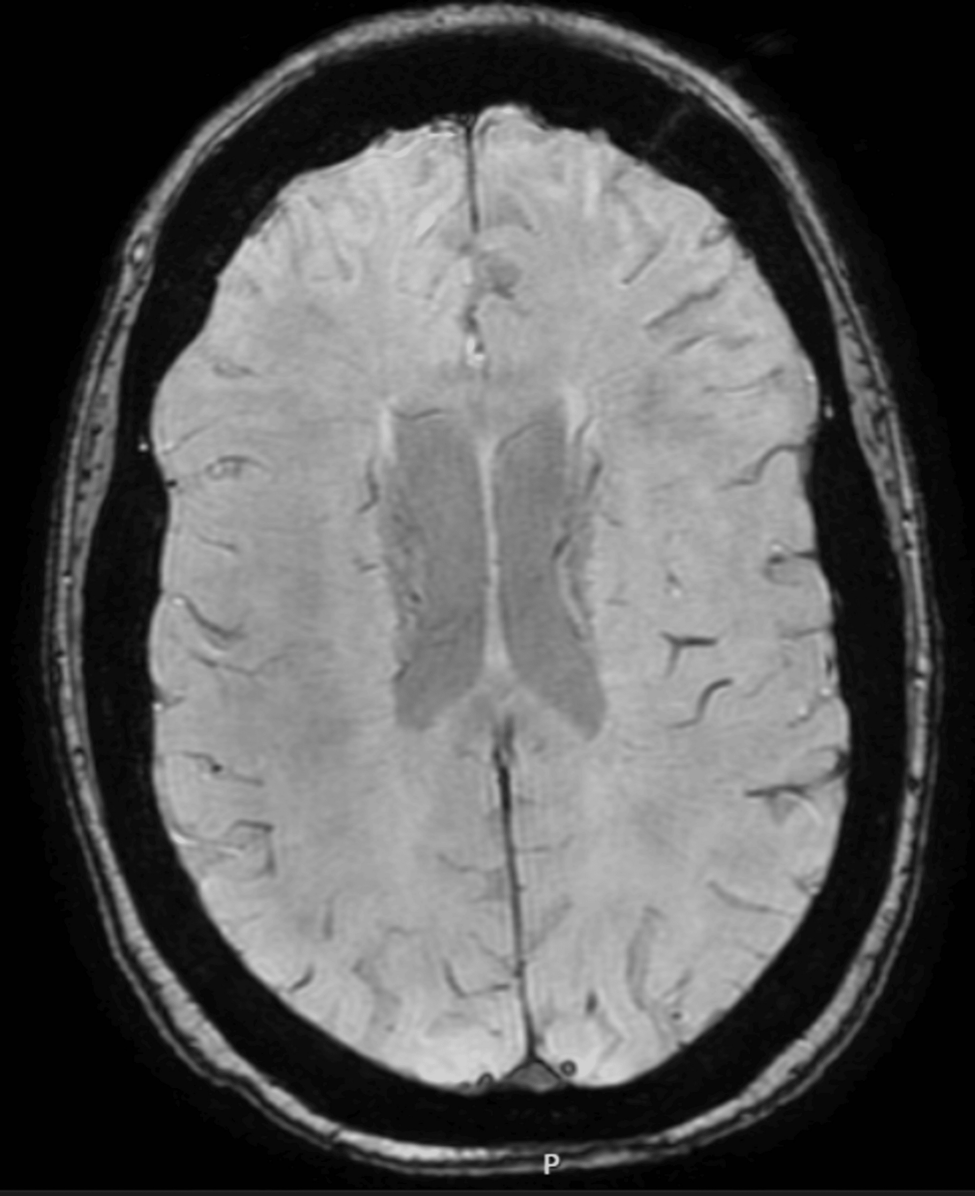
MRI brain with no evidence of intracranial lesion or pathology MRI: magnetic resonance imaging
